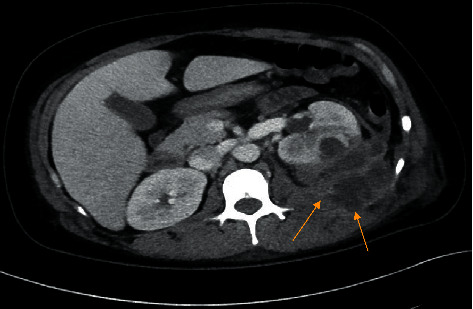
Computed tomography (CT) scan of the abdomen and pelvis showing left kidney and extensive expansion into the left retroperitoneum, psoas muscle, and possibly even the left posterior abdominal wall with multiple loculated abscesses.
